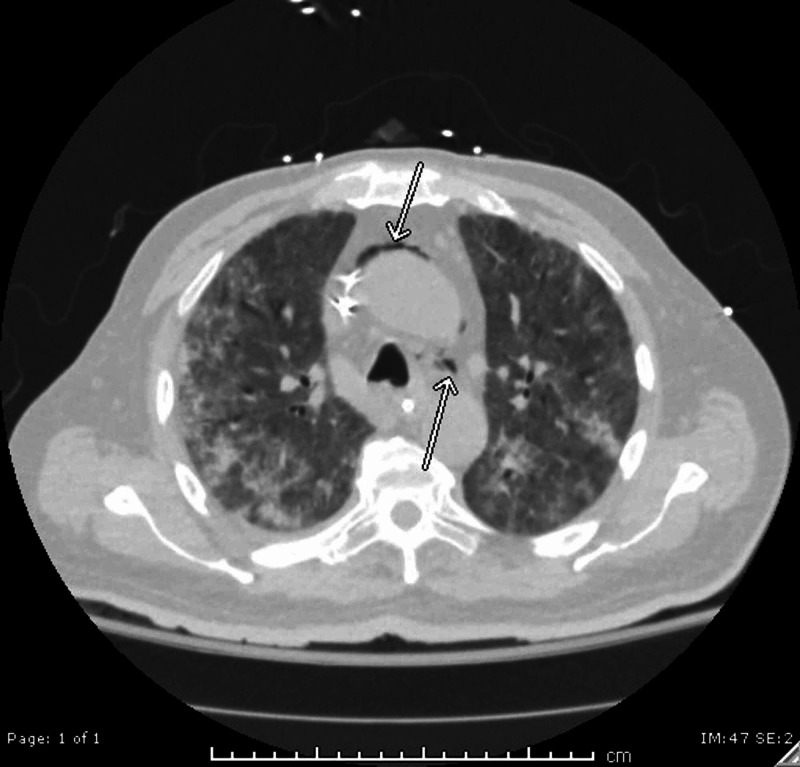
A lung window CT image of a superior portion of the thorax showing pneumomediastinum (arrows).
